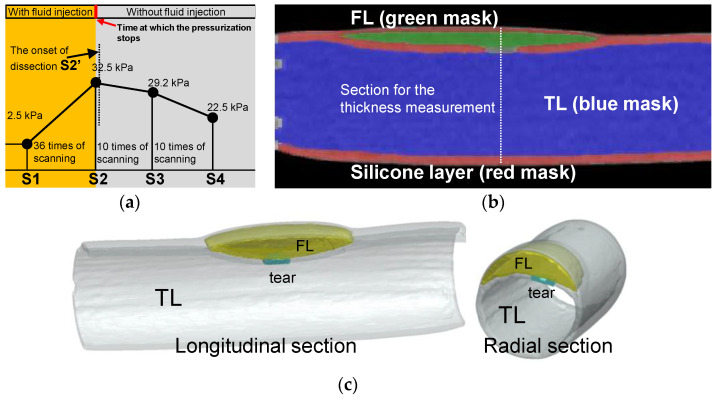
Experimental process of CT scanning and image reconstruction. (a) Pressure setting and the protocol of the CT scanning. (b) Reconstructed model of the longitudinal section from CT images at time point S2. (c) 3D reconstructed model of the longitudinal and cross-sectional view at time point S2′.
